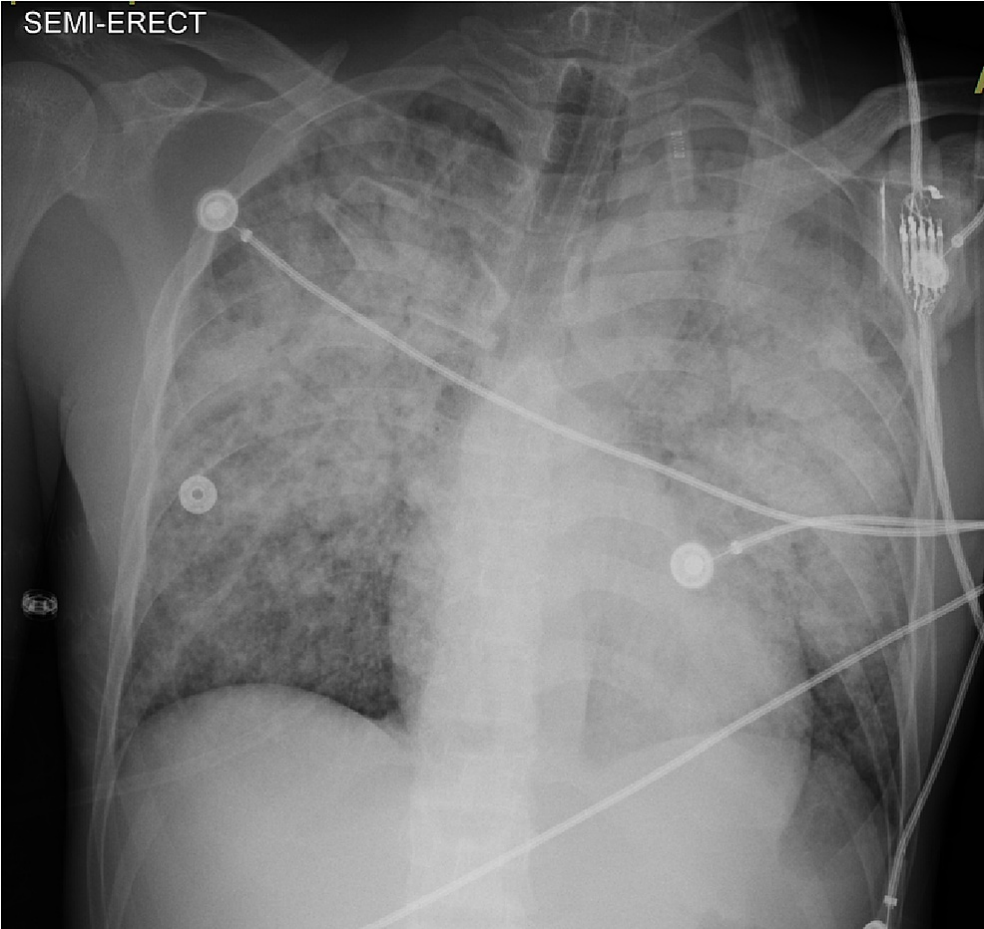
Chest x-ray showing diffuse bilateral opacities in the upper lungs.
